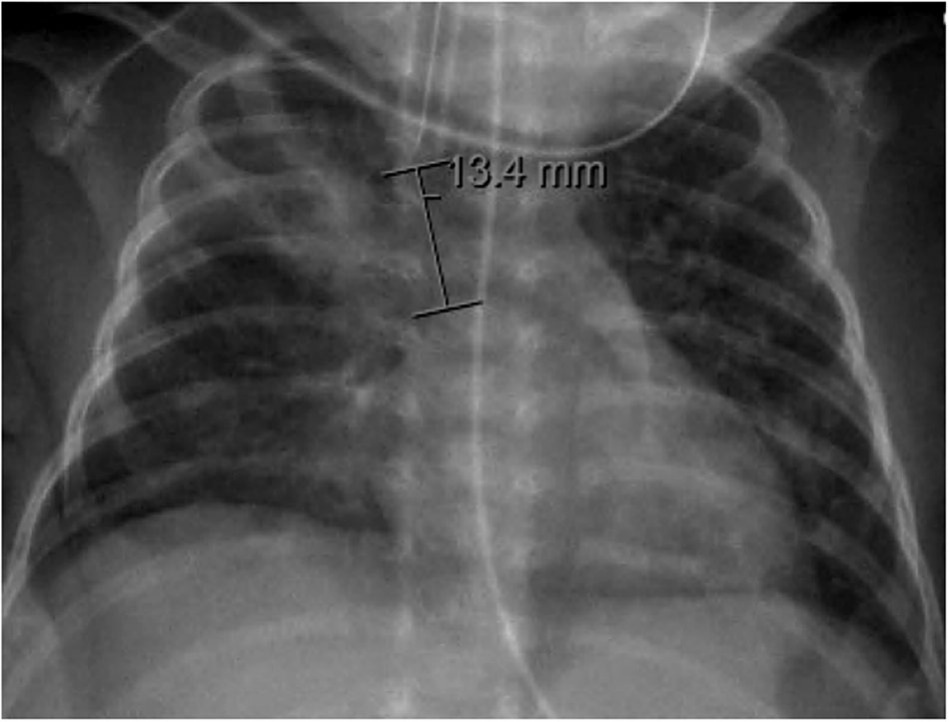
Chest radiograph images of neonates diagnosed with ventilator-associated pneumonia based on CDC guidelines. This radiograph was used to diagnose a ventilator-dependent 5-week-old, 27-week GA male with *Klebsiella pneumoniae* and *Staphylococcus aureus* pneumonia. CDC Centers for Disease Control and Prevention, GA gestational age.
